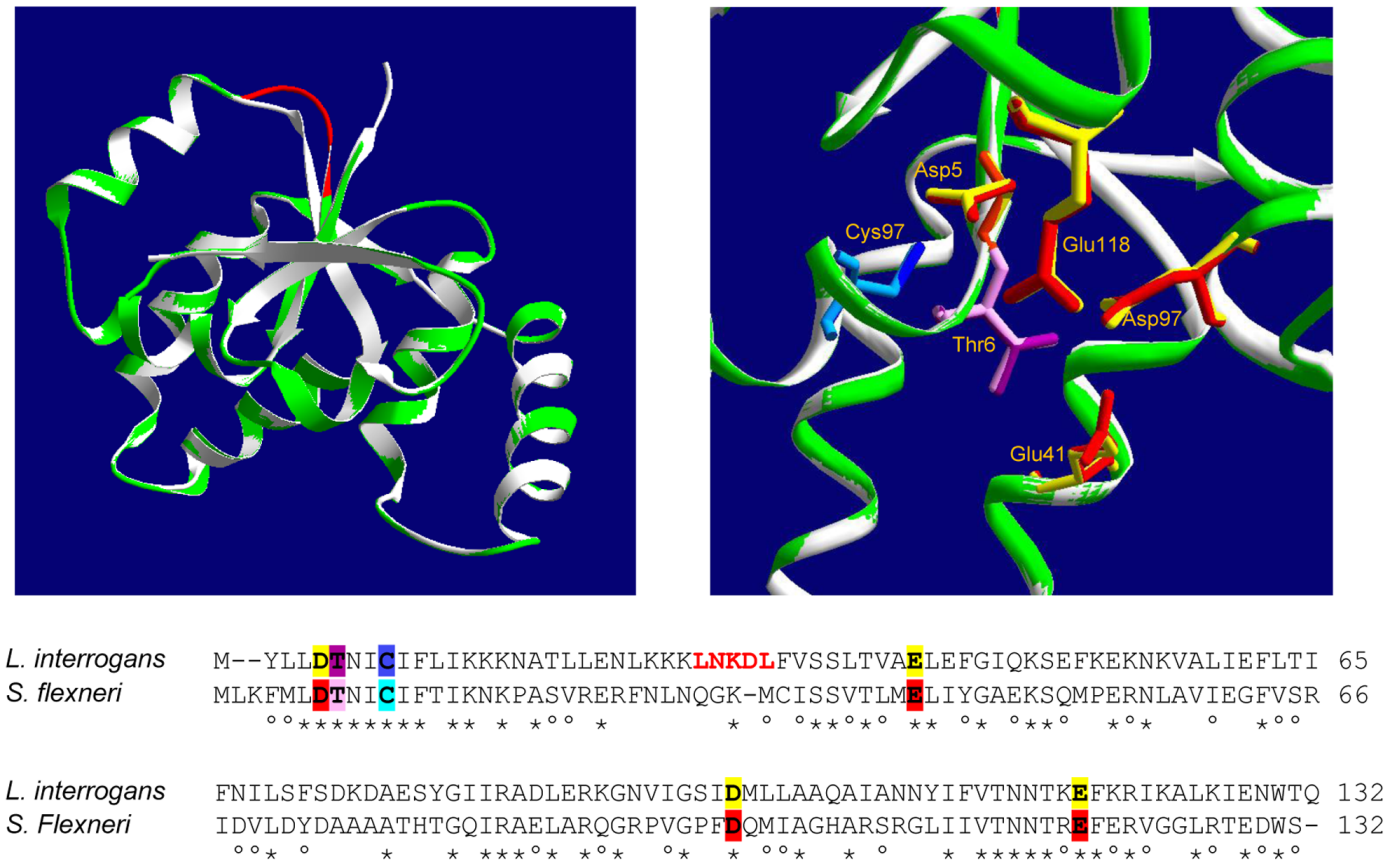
3D model of leptospiral VapC closely matches the experimental X-ray structure of *Shigella*'s VapC. Alignment of the sequences of VapCs from *S. flexneri* and *L. interrogans* is shown. On the left panel, the structure model (ribbon) of VapC from *L. interrogans* (green) was superimposed to the VapC template from *S. flexneri* (white) (PdB: 3TND-C). The green regions in the target appear almost identical to the template, while the red region does not correspond. The amino acids composing the red region are written in the same color in the sequence alignment. On the right panel, superimposition shows the perfect matching of the conserved threonine and the four acidic residues responsible for coordinating metal ions in the catalytic site, and the cysteine involved in dimerization, positioned in the neighborhood of the catalytic site. These six residues are numbered in the structure according to leptospiral VapC sequence and colored as highlighted in the alignment.
